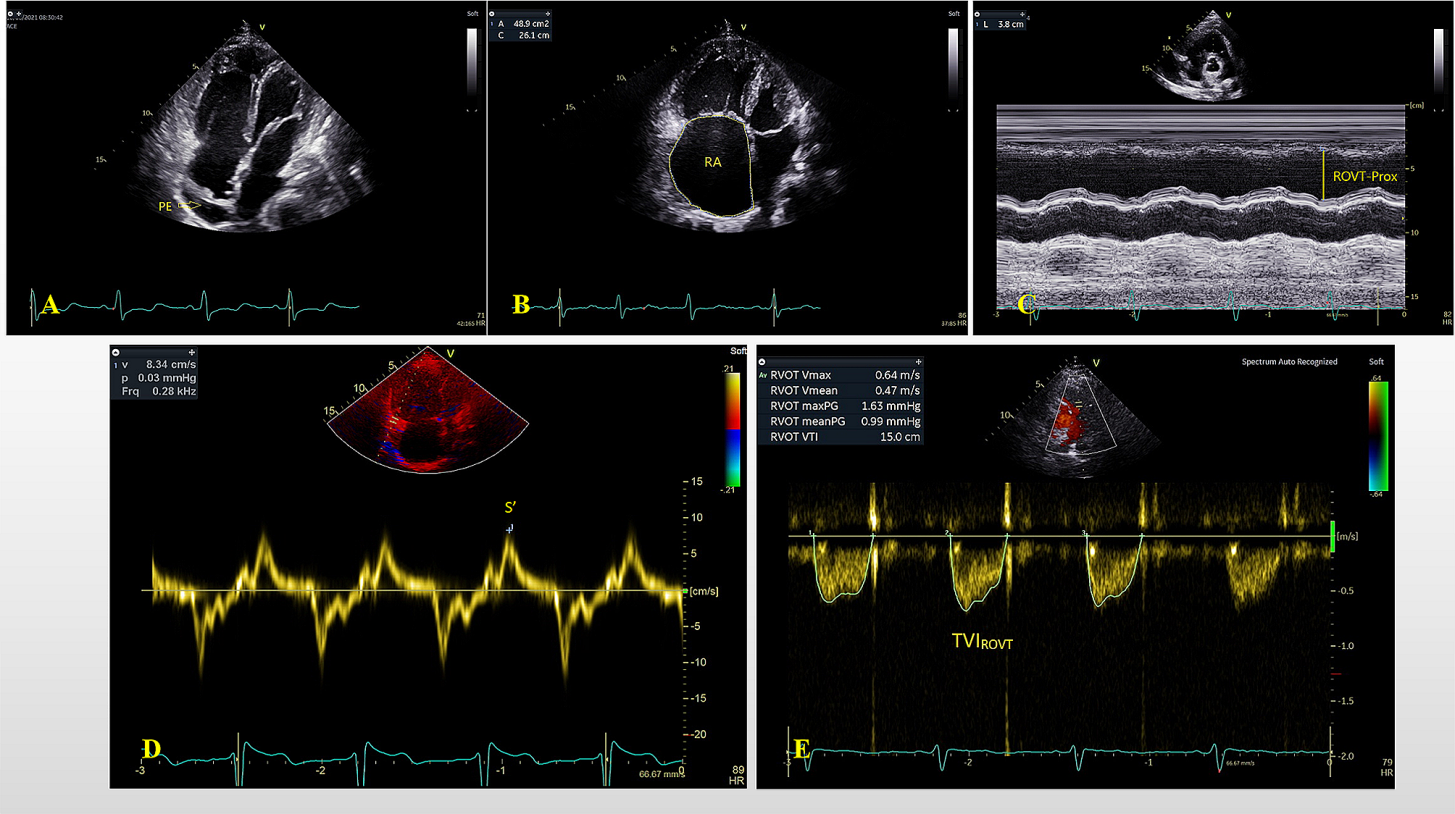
Five echocardiographic parameters to be measured in echocardiographic scoring system. A. pericardial effusion (PE) B. Right atrial (RA) area C. Right ventricular outflow tract proximal diameter (RVOT-Prox) D. Systolic annular tissue velocity of the lateral tricuspid annulus (S’) E. The velocity time integral of the right ventricular outflow tract (TVI_RVOT_)
